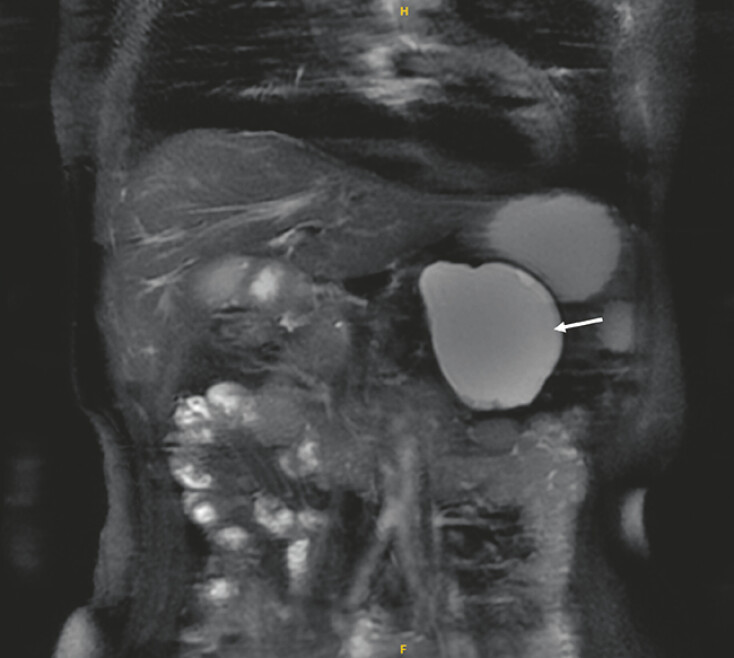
Magnetic resonance imaging scan showing a pancreatic pseudocyst (arrow) located in the tail of the pancreas.
